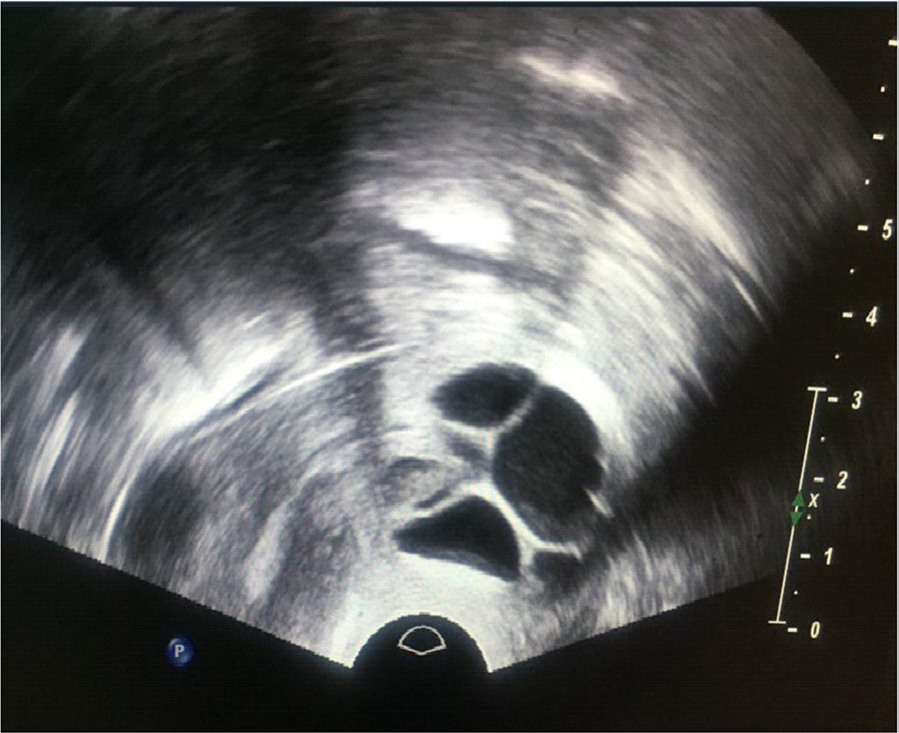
Transvaginal ultrasound: sagittal view showing anechoic cysts in the cervix
